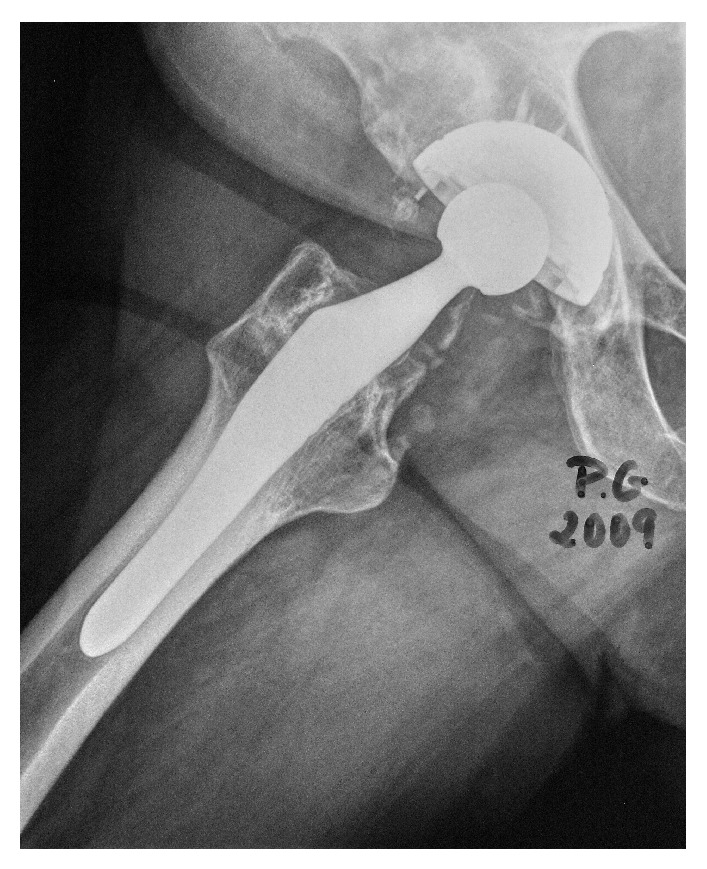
X-ray control of patient in Figures 2 and 3, after 4 y follow-up, after changing polyethylene and fulfilling with bone graft the acetabulum osteolysis. No change of original implant.
